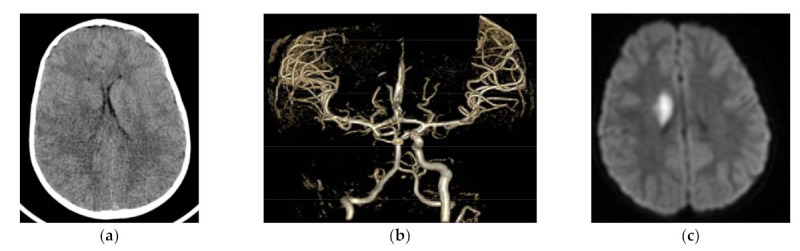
Acute focus of LACI (lacunar anterior circulation infarct) in right caudate nucleus: (a) CT (computed tomography) six hours after the onset—very subtle density blurring at the level of right caudate nucleus; (b) CTA (computed tomography angiography)—occlusion of right ICA (internal carotid artery) with sustained flow in MCA (middle cerebral artery) and ACA (anterior cerebral artery); (c) MRI DWI (diffusion weighted imaging in magnetic resonance)—restricted diffusion in right caudate nucleus region.
